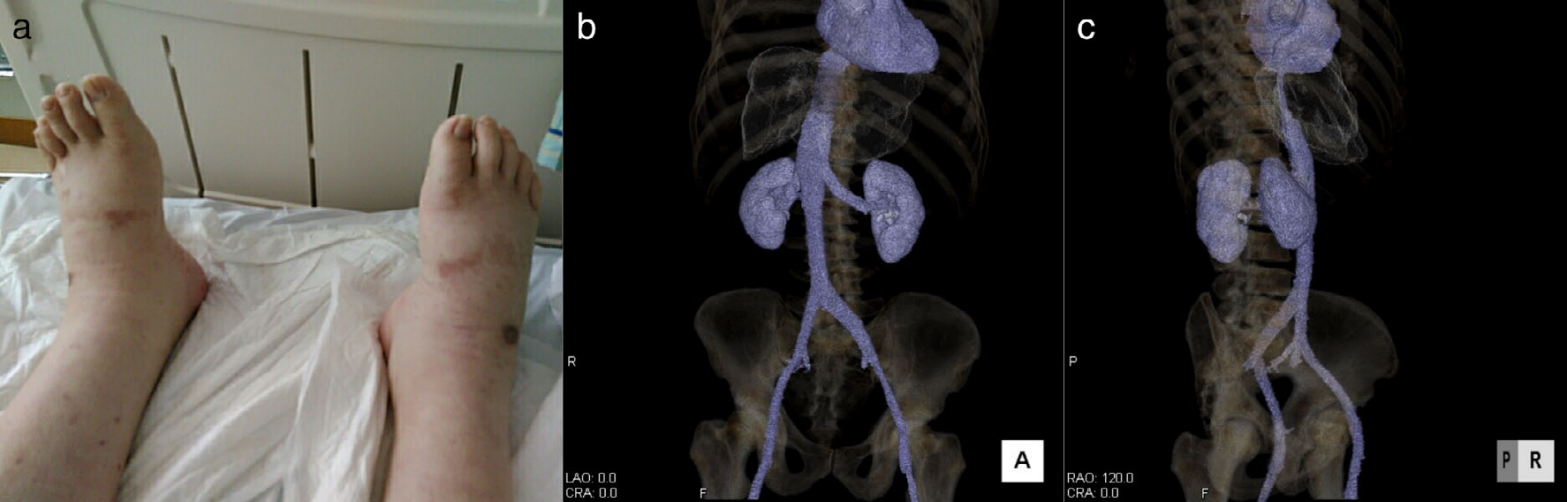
Symptoms associated with pseudocirrhosis and three‐dimensional computed tomography (CT) findings of the inferior vena cava. (a) Marked leg edema; (b) and (c) Stenosis of the inferior vena cava near the liver
